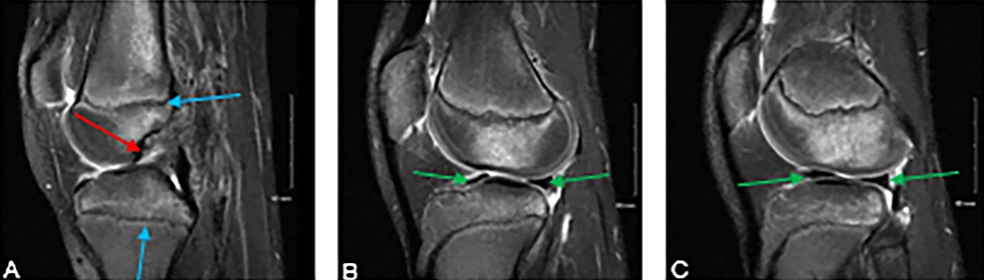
T-2 weighted MRI sequences of the left knee demonstrating an isolated ACL tear (A) (red arrow). Medial (B) and lateral (C) menisci appear to be intact (green arrows). The open physis are identified with blue arrows.
